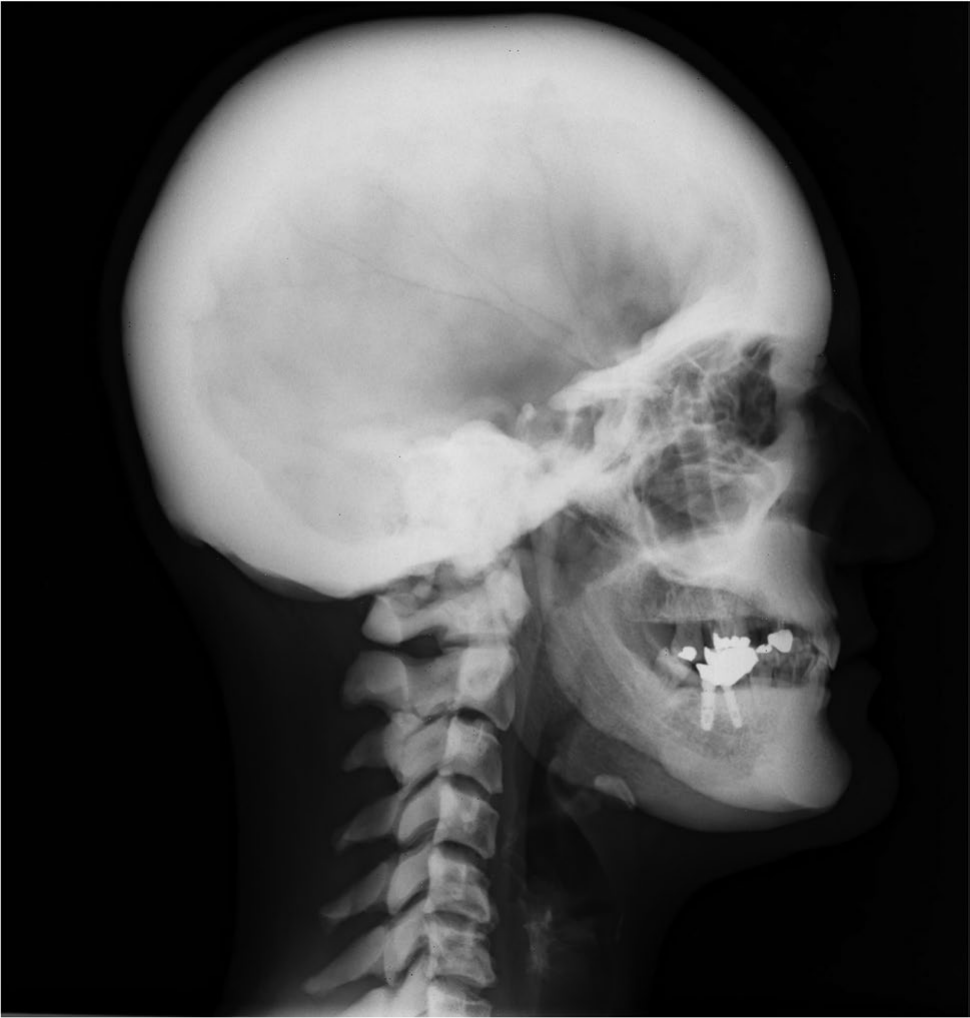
X-rays showing severe osteosclerosis of the skull, particularly at the base. Written informed consent was obtained from the patient for publication of the radiograph7
